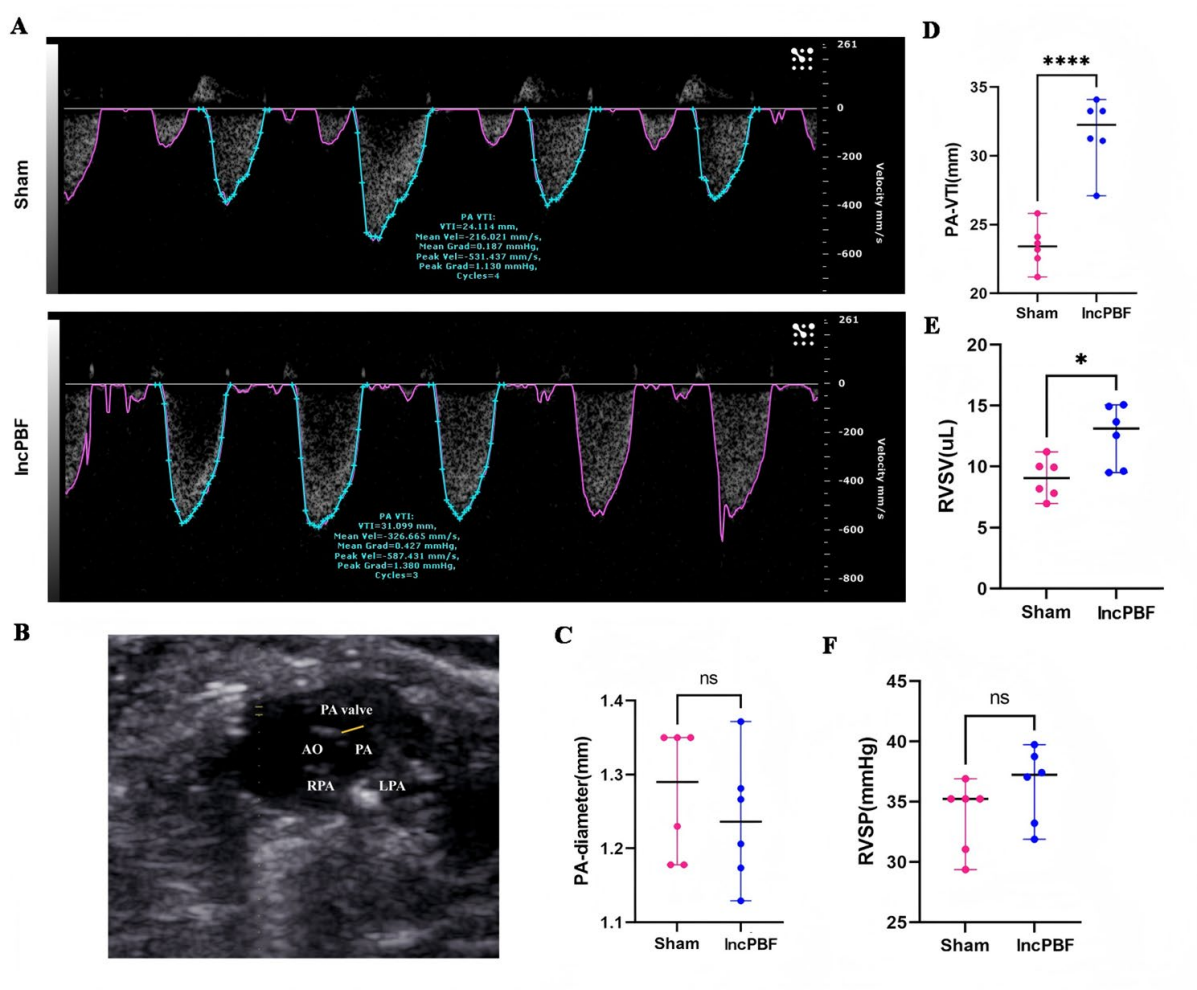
Neonatal shunt generates IncPBF. A Representative blood flow imaging at the pulmonary valve. B Representative echocardiography at the pulmonary valve. Blue line indicates the PA diameter. C Quantification of the PA diameter. D Quantification of PA-VTI. E Quantification of RVSV. F Quantification of RVSP
